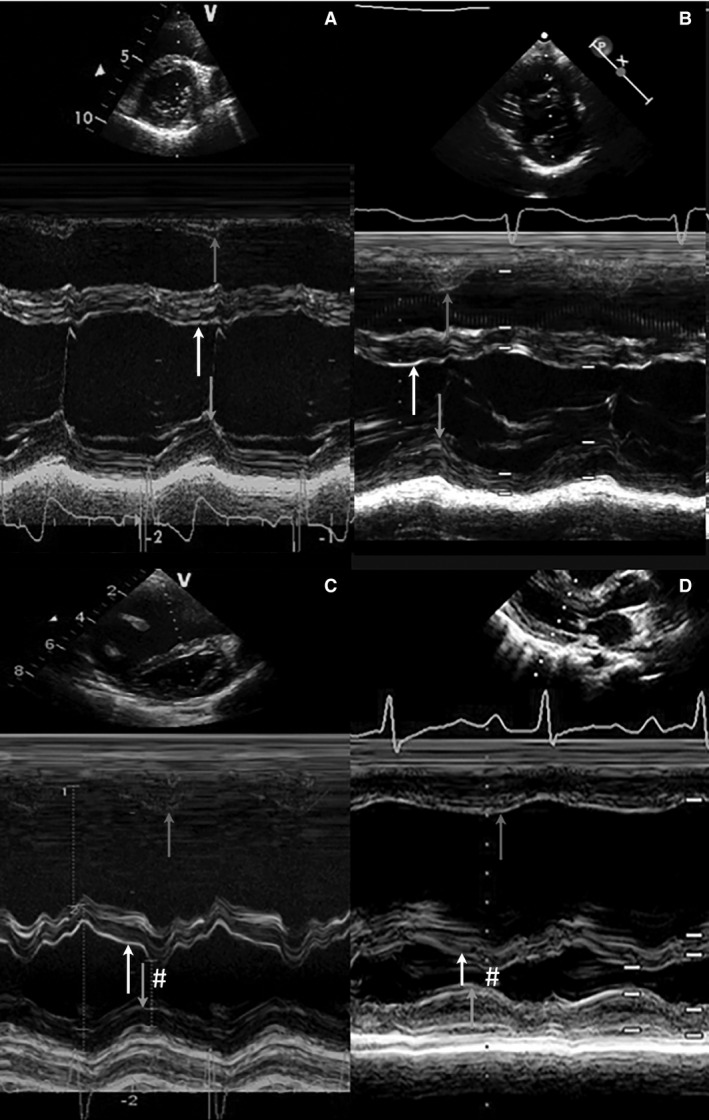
M‐mode cross sections. Four representative examples of m‐mode cross‐sectional parasternal short or long axis at the level of MV annulus are shown. The time to peak radial motion of right ventricular free wall, septum, and left ventricular posterior wall are depicted with arrows. (A) control, (B) pulmonary stenosis and (C and D) idiopathic pulmonary arterial hypertension. Abnormal septal motion in iPAH patients at the beginning of LV diastole is marked with # (C and D).
